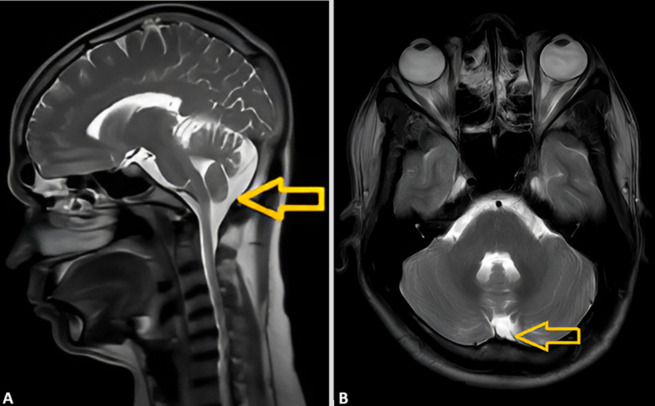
magnetic resonance image scans of the brain (T2-weighted) with; A) the sagittal, and B) axial planes show mega cisterna magna in the retro-cerebellar region as a fluid-filled space, marked with yellow arrows
